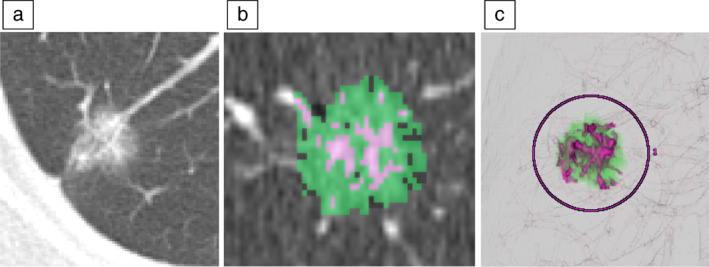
High‐resolution computed tomography (CT) (a). Ground‐glass opacities (GGO) (green) and solid (purple) regions analyzed with the Synapse Vincent software program (b) and the 3D image used for the analysis (c)
